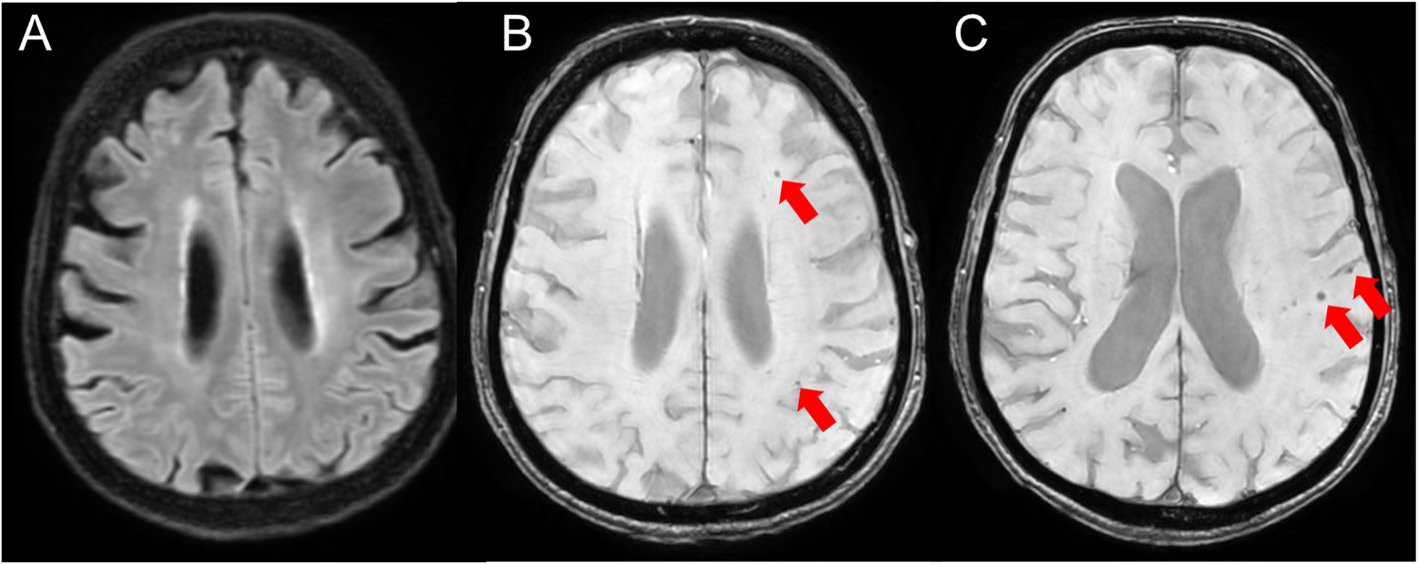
Day 9: blooming of Microbleeds and Disappearance of the HARM Sign. A Non-enhanced FLAIR MRI sequence shows no more evidence of the HARM sign 7 days after the acquisition of the images depicted in Fig. 2. B and C SWAN MRI sequence shows the appearance of multiple hemosiderin spots suggestive of microbleeds (red arrows). FLAIR indicates fluid attenuated inversion recovery; HARM, hyperintense acute reperfusion marker; MRI, magnetic resonance imaging; SWAN, susceptibility-weighted angiography
